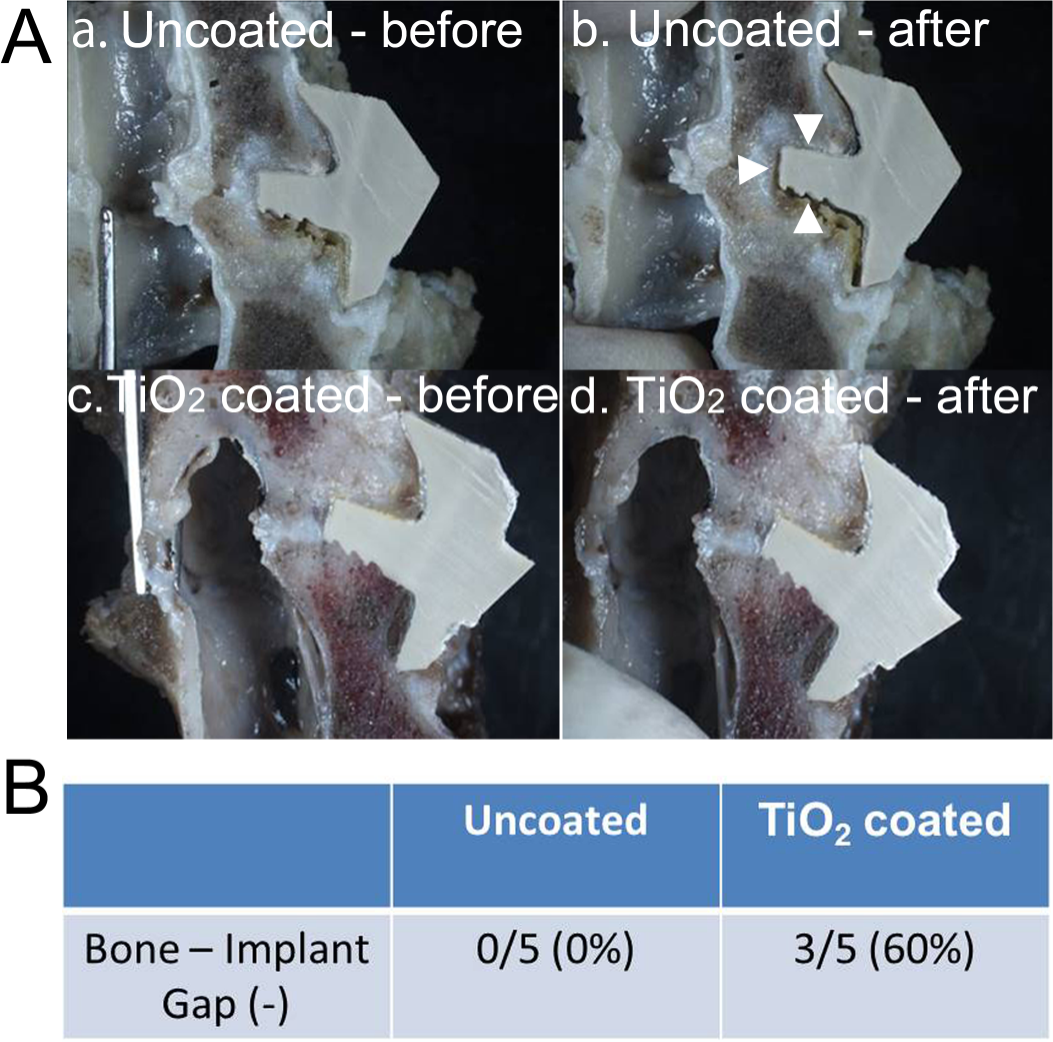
(A) The μ-CT images. (a) Uncoated PEEK. Bone cysts and osteolytic lesion were observed surrounding the PEEK implant (black arrowheads). (b) TiO_2_-coated PEEK. An intervertebral bony bridge was observed (white arrowheads). (B) The bony union rate of the PEEK implants based on the μ-CT finding.
